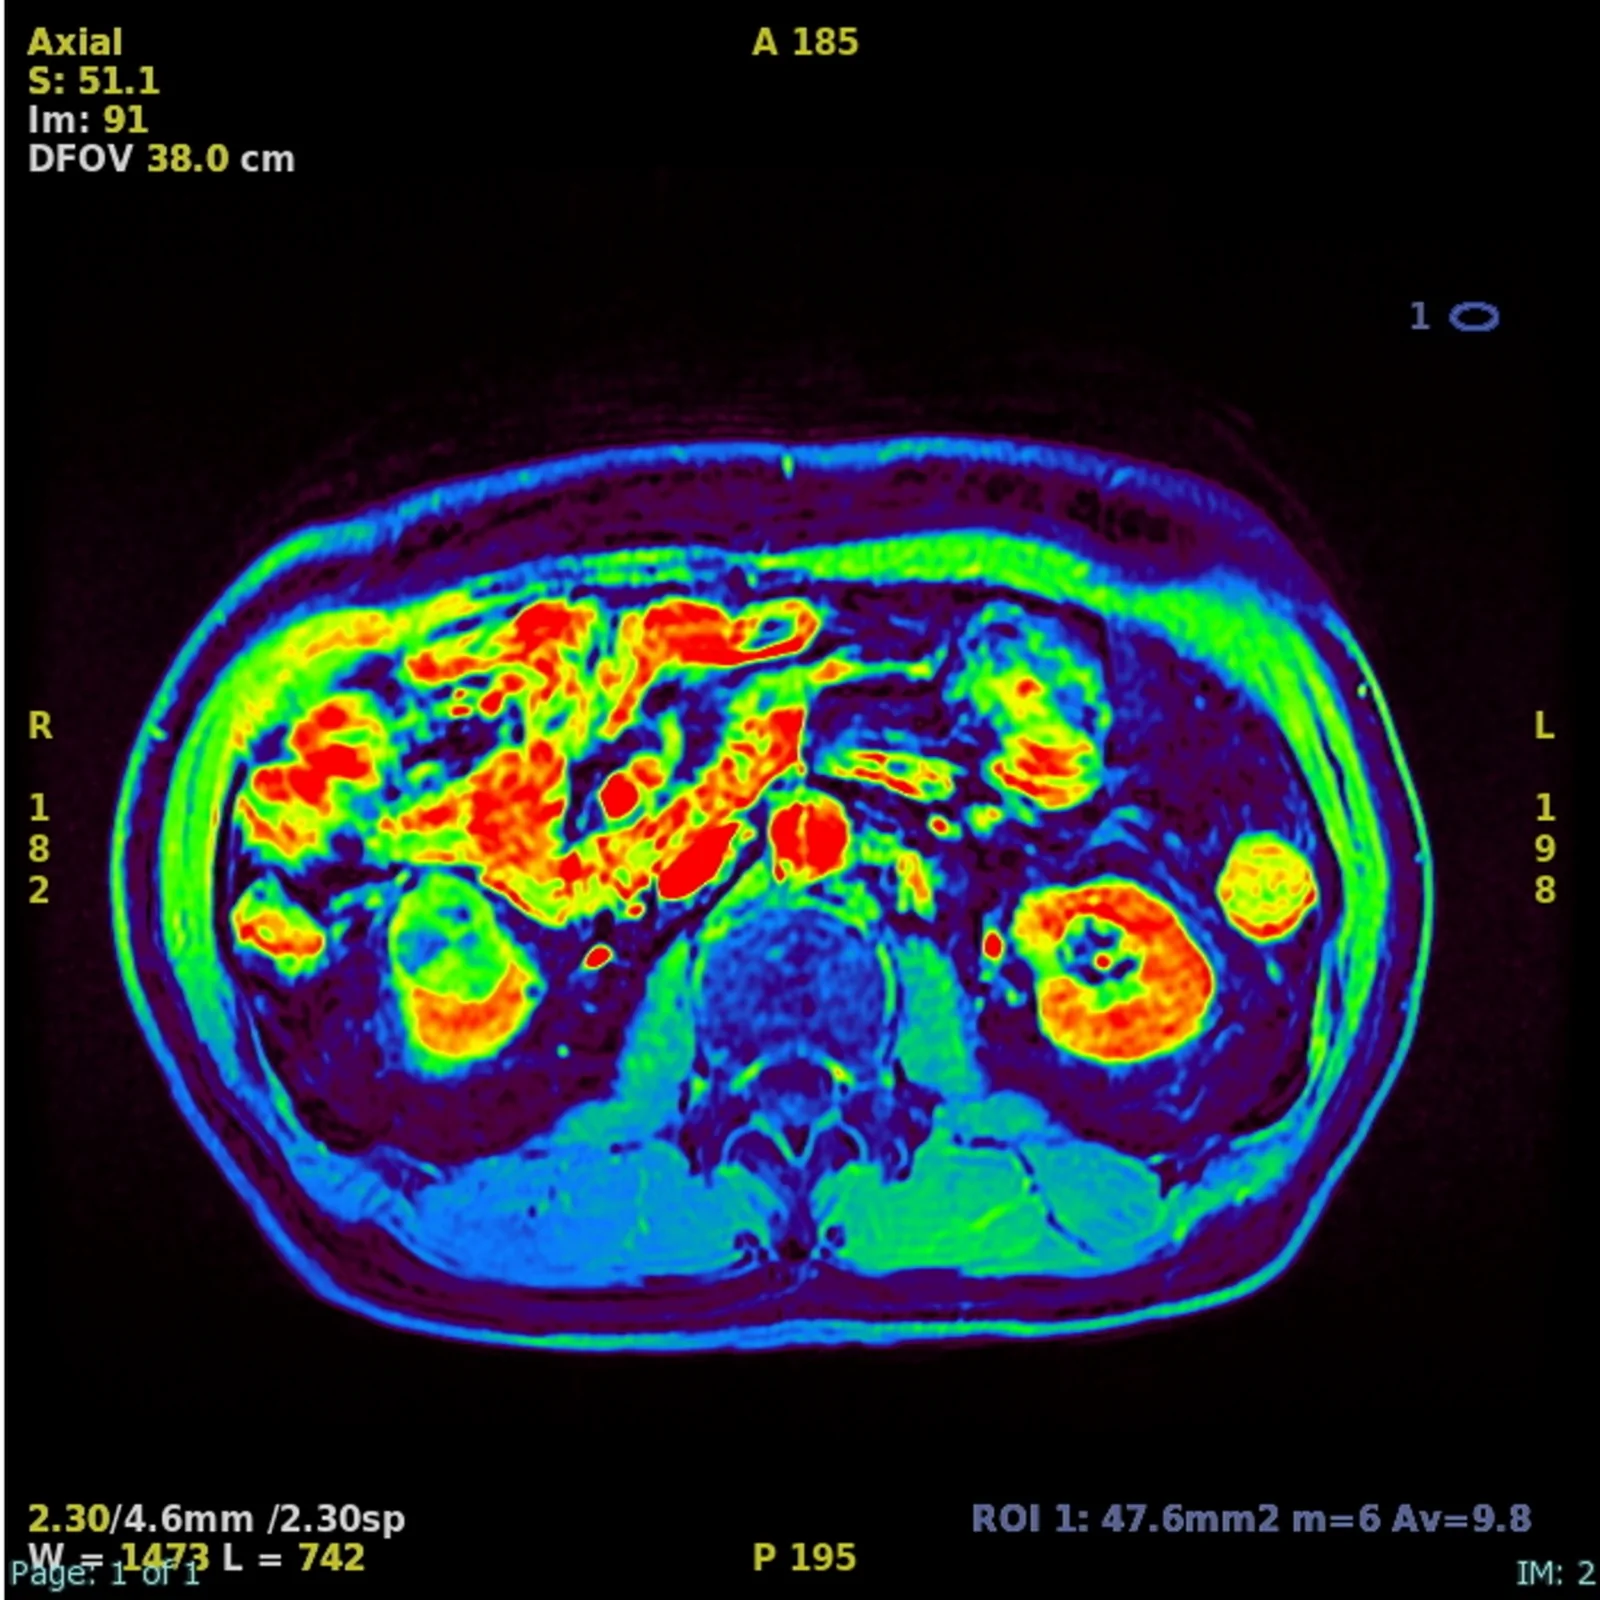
Dual-energy CT iodine map showed a heterogeneous mass at the right lower renal pole, characterised by hyperattenuating cystic content and faint iodine uptake within the mural component
